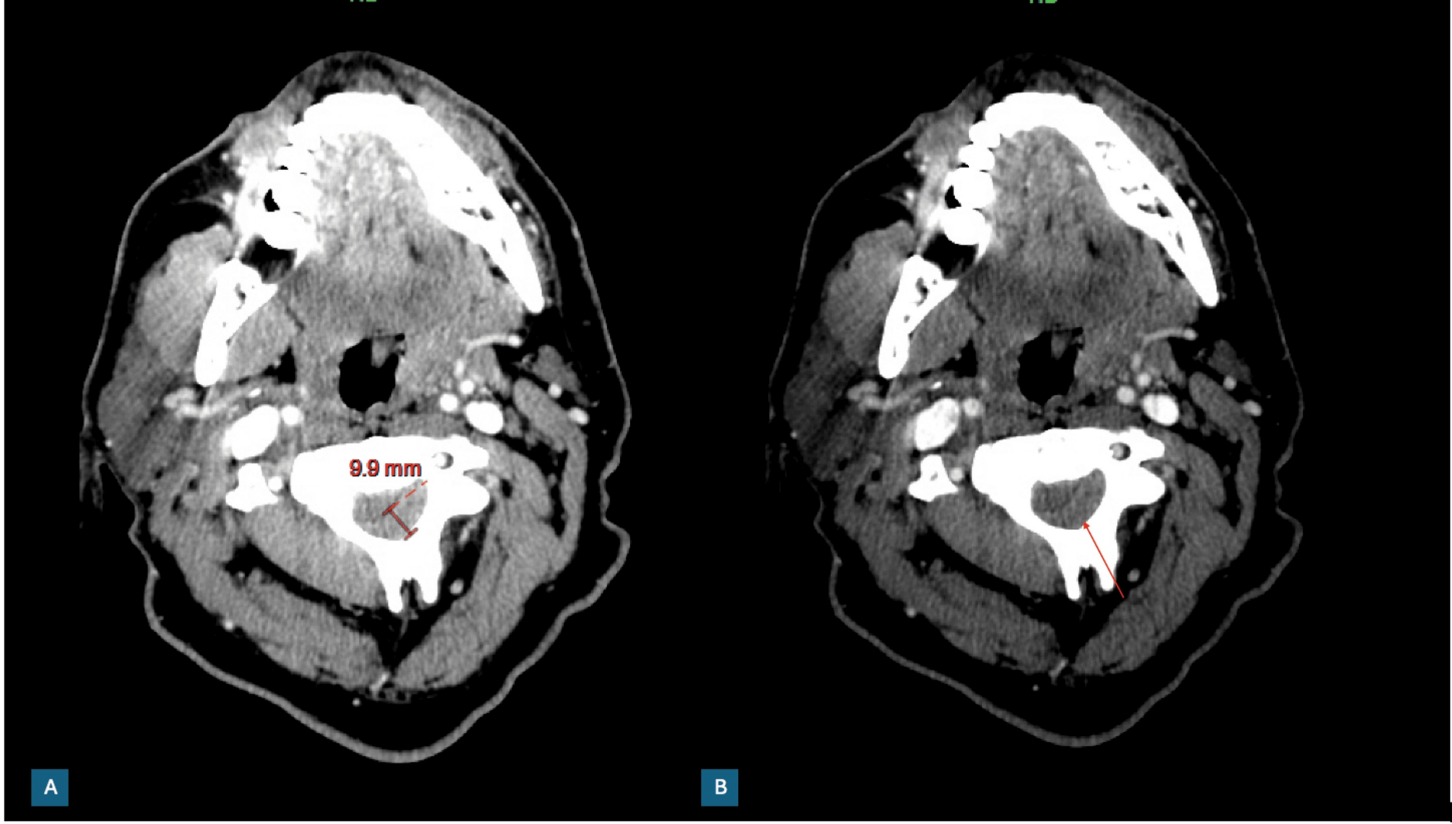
Key image from the emergent CT angiography (CTA) head, demonstrating abnormally high attenuation material in the central canal. (A) Measurement of the high attenuation material up to 1 cm in measurement. (B) An arrow pointing to the area of material.
